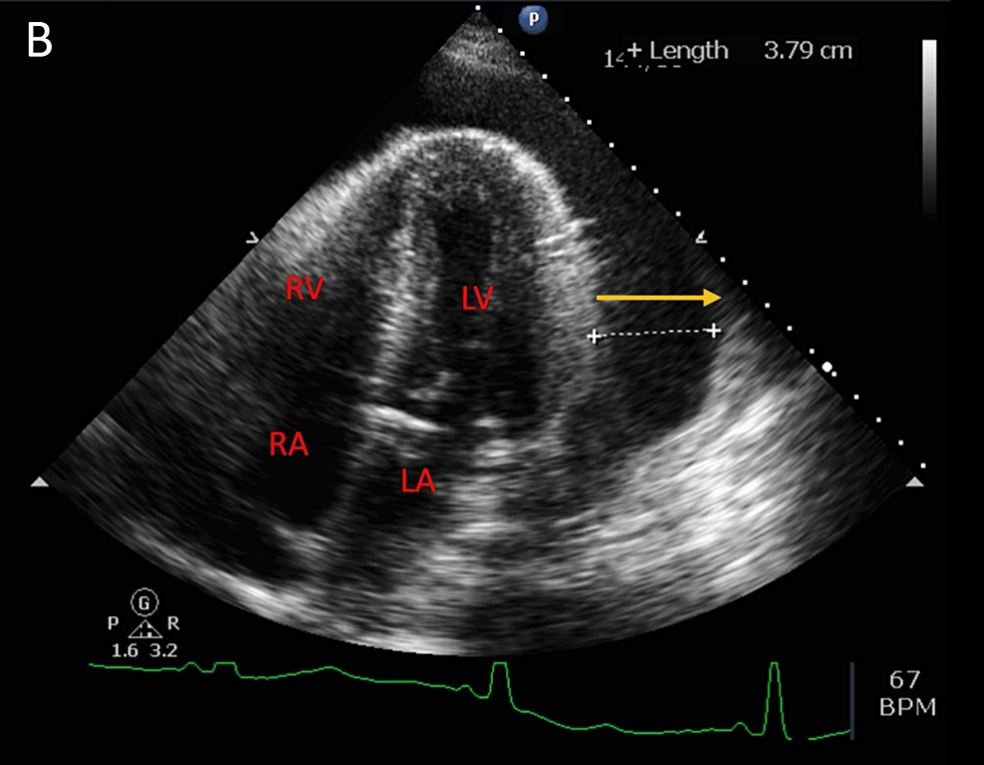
Transthoracic echocardiogram four-chamber view depicting large volume pericardial effusion (yellow arrow). LV: left ventricle; LA: left atrium; RV: right ventricle; RA: right atrium.
